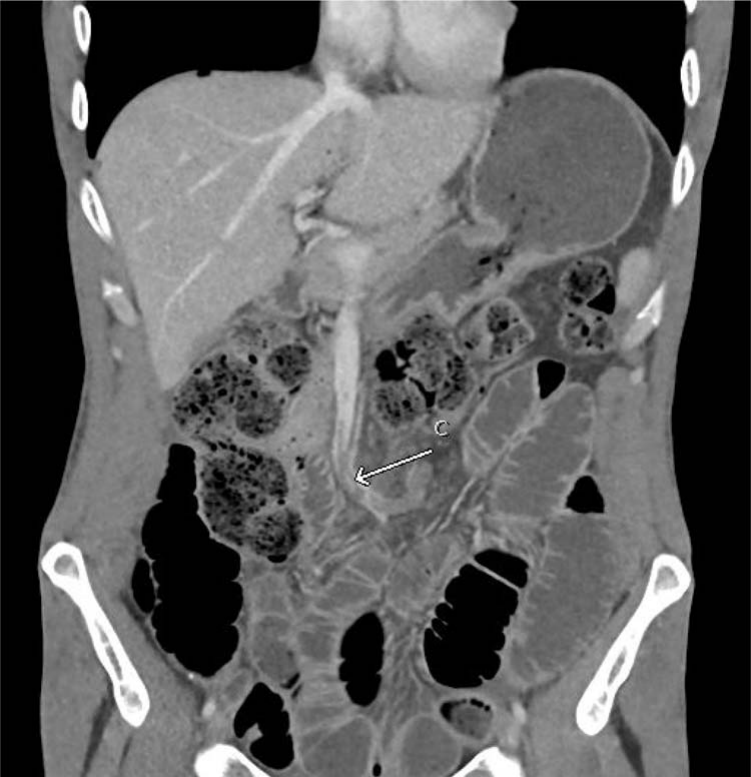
Repeat CT abdomen pelvis with contrast in the portal venous phase showed (C) filling defect in the superior mesenteric vein. Multiple dilated small bowel loops without a clear transition point were also evident, suggestive of ileus.
